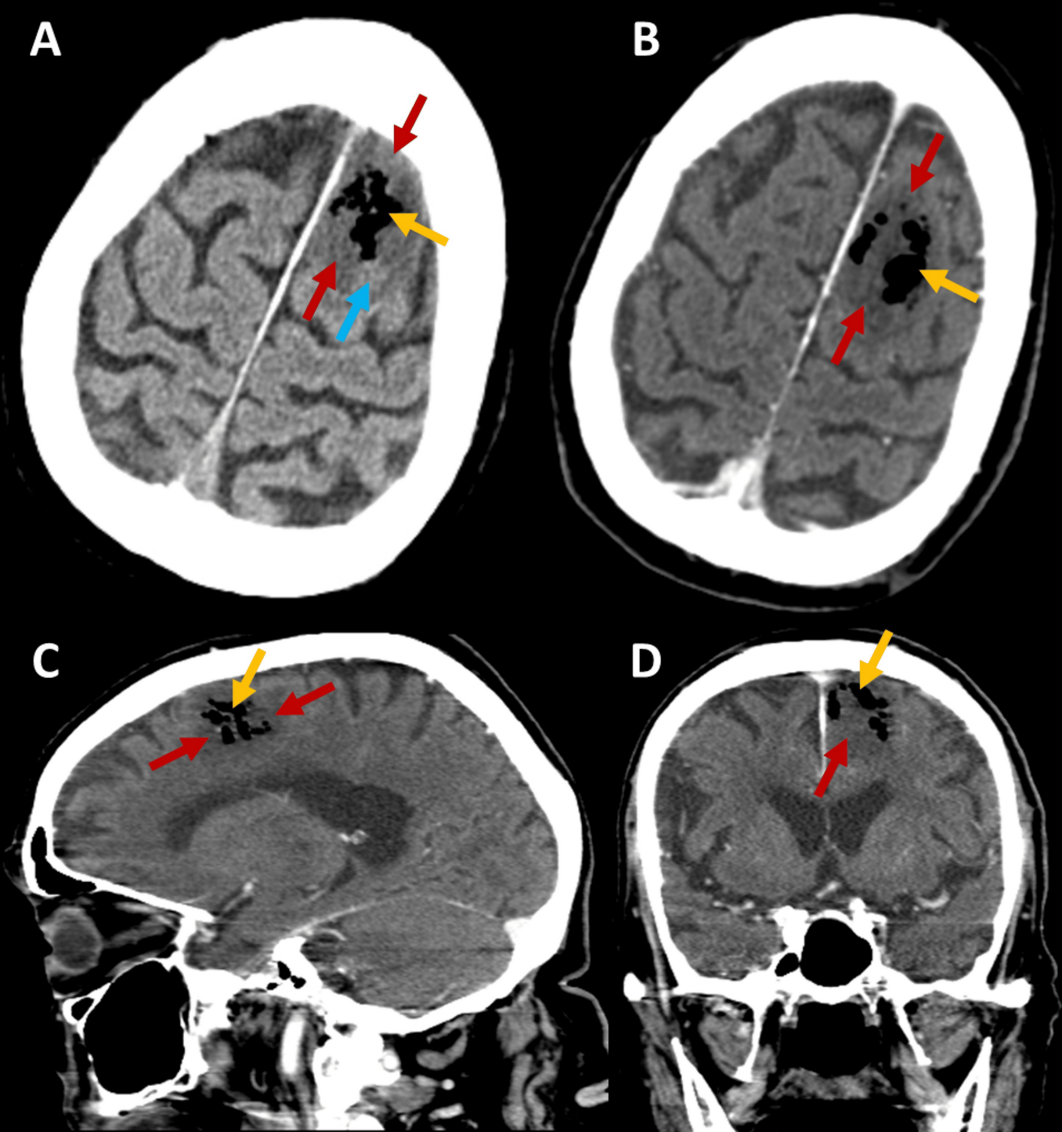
Brain CT scan images with contrast (B-D) and without contrast (A), revealing a cortico-subcortical hypodense lesion in the left frontal lobe (red arrows) with gaseous content (yellow arrows), shown in axial (A-B), sagittal (C), and coronal (D) planes. The blue arrow indicates an area of higher spontaneous density within the lesion.
